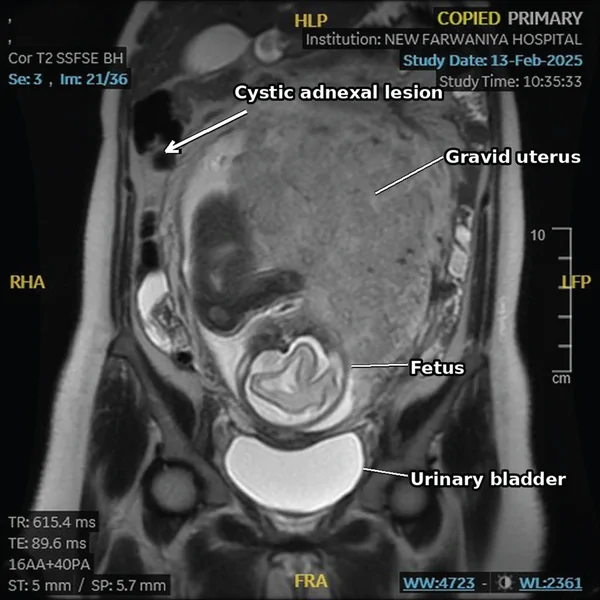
Pelvic MRI, adjacent coronal T2‐weighted slice from the same acquisition, confirming the adnexal lesion (white arrow) on two contiguous sections. Annotations as in Figure 2.
